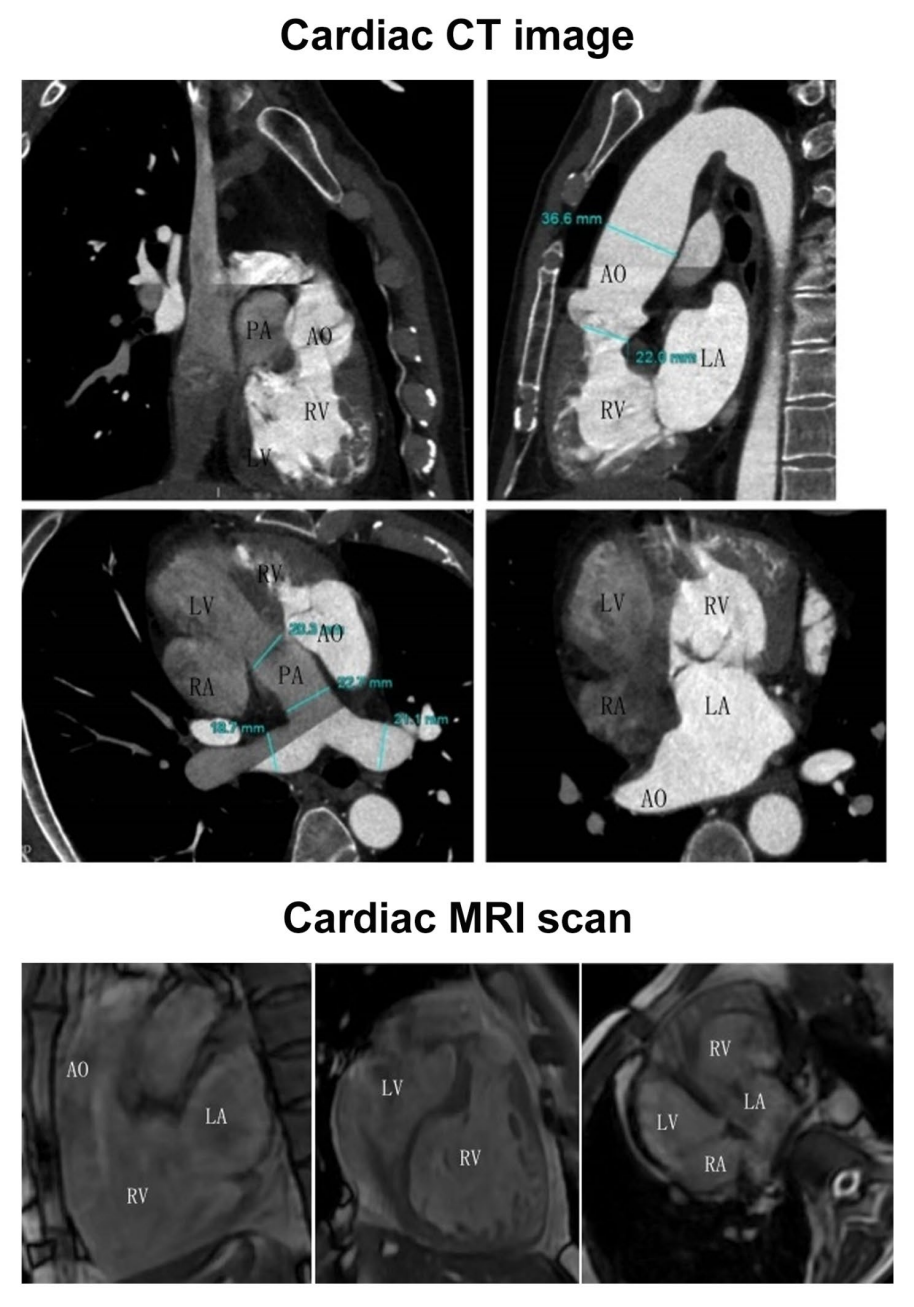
Cardiac CT and MRI image (PA = pulmonary artery, AO = aorta, LA = left atrium, RA = right atrium, LV = left ventricle, RV = right ventricle). Both CT and MRI images showed that the aorta was connected to the right ventricle and the pulmonary artery was connected to the left ventricle. The myocardium in the anatomical right ventricle was thicker than that in the anatomically normal right ventricle, and the trabecular myocardium in the anatomical left ventricle was less
